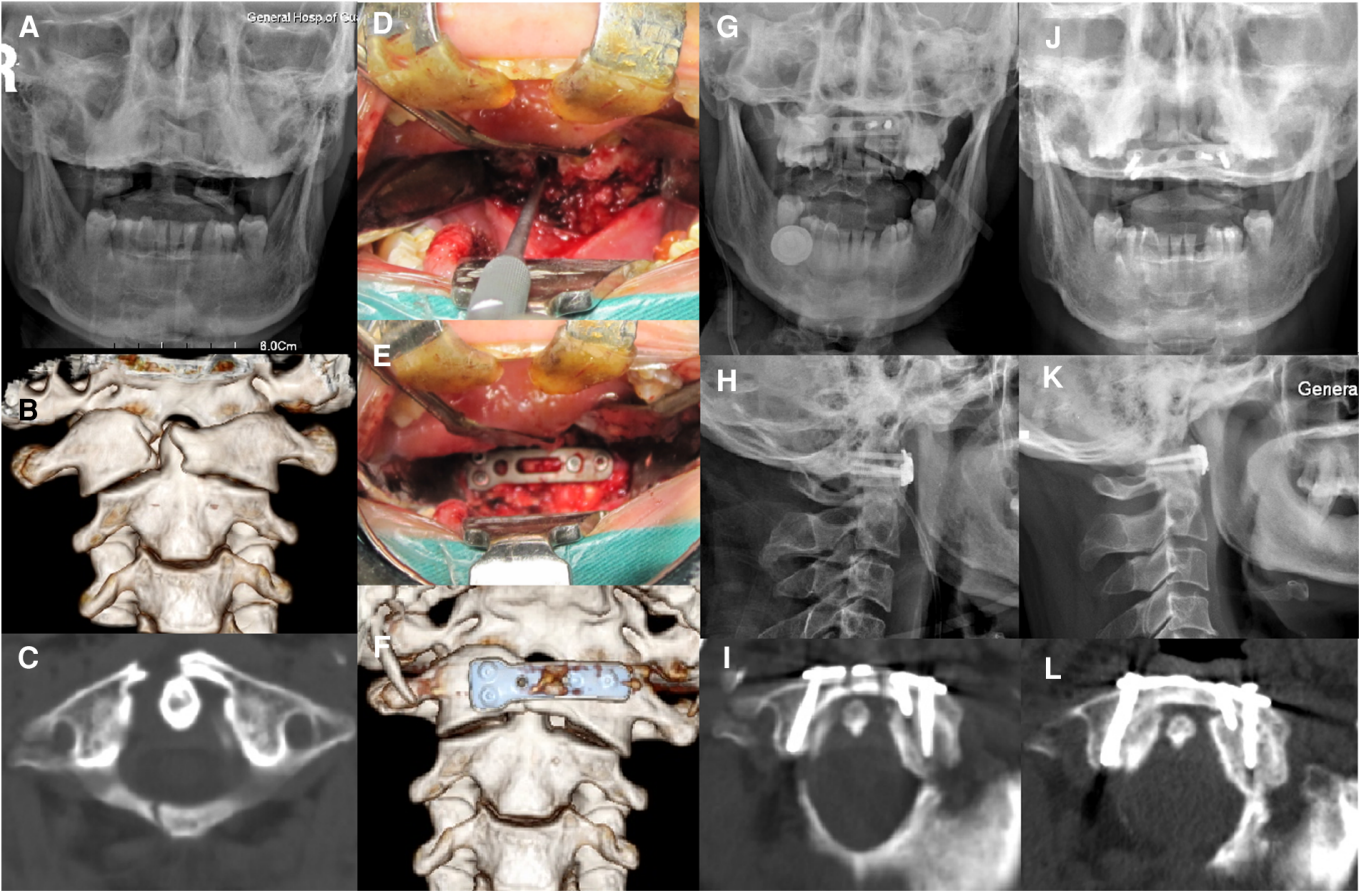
A 47-year-old female with combined fractures of the anterior and posterior atlantal arches was treated by transoral anterior C1-ring osteosynthesis using the novel reduction plate. (A) Preoperative open-mouth x-ray imaging showed displacement of the lateral masses. (B,C) The reconstructed images in the coronal and axial CT scan revealed fractures through the right side of the anterior and posterior arches of the atlas with displacement of the lateral mass. (D,E). Intraoperative photographs of an anterior arch fracture before and after fixation. (F) The reconstructed images after surgery showed optimal plate location. (G,H). Postoperative open-mouth and lateral x-ray imaging identified the relatively good C1–C2 alignment. (I) An axial CT image after surgery revealed reduction of the anterior arch fracture and adequate screw placement. (J,K) Open-mouth and lateral x-ray images at 6 months after surgery showed no loosening of the plate or screws. (L) An axial CT image at 6 months after surgery revealed solid bone fusion.
